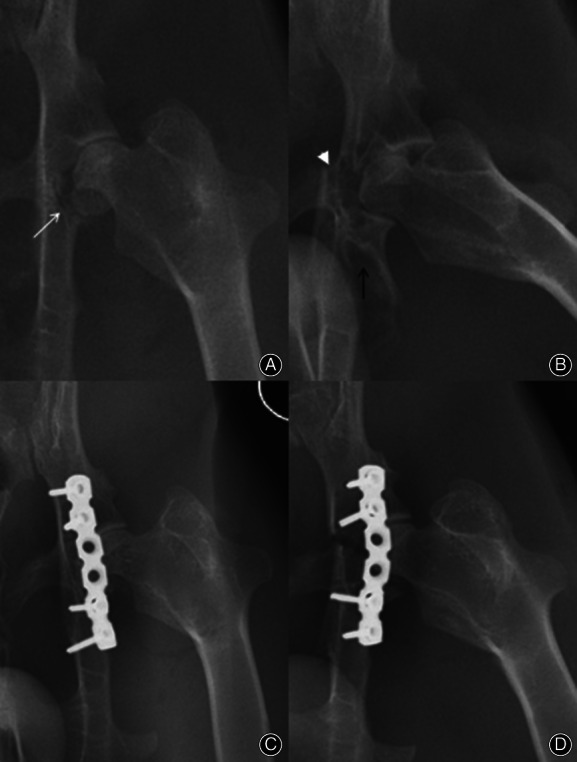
Representative radiographs before and after fixation: (A) and (C): anteroposterior (AP) radiographs of a pure dorsal wall fracture (white arrow) of acetabulum before and after ORIF; (B) and (D): anteroposterior (AP) radiographs of a transverse fracture (white arrowhead) presented with an enlarged dorsal fragment (black arrow) that involved all of the caudal part of acetabulum before and after ORIF.
